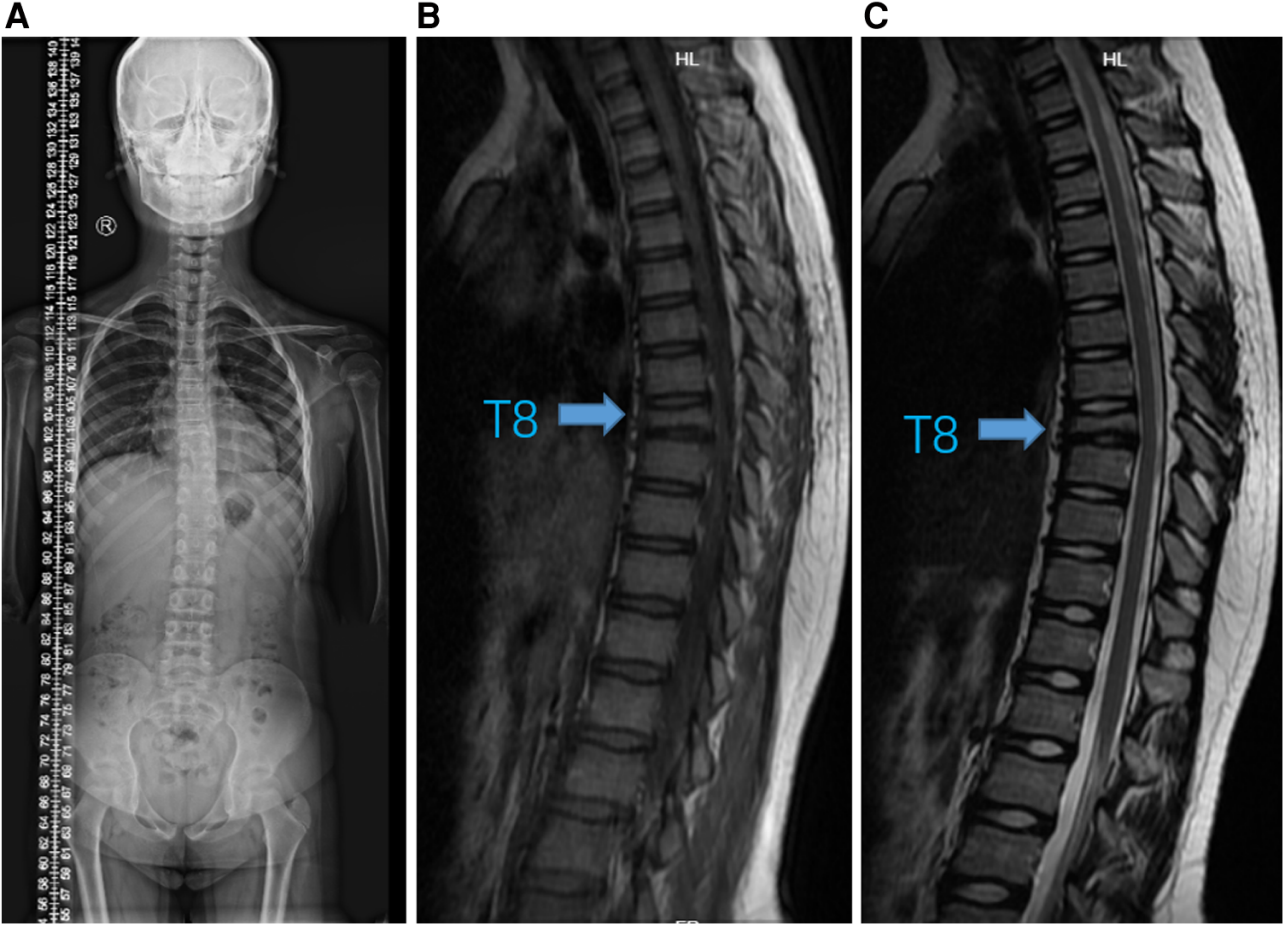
Imaging changes after six months of therapy. (A) Standing spinal x-ray. (B) T1-weighted and (C) T2-weighted MRI images showed that T8 vertebra remained stable without progression (arrow).
